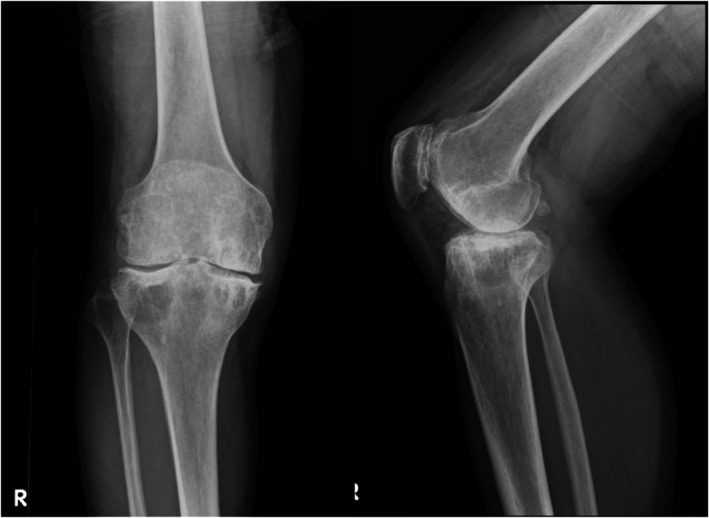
Plain radiographs 19 months after the second surgery. Anteroposterior and lateral views showing no progression of radiographic joint destruction.
